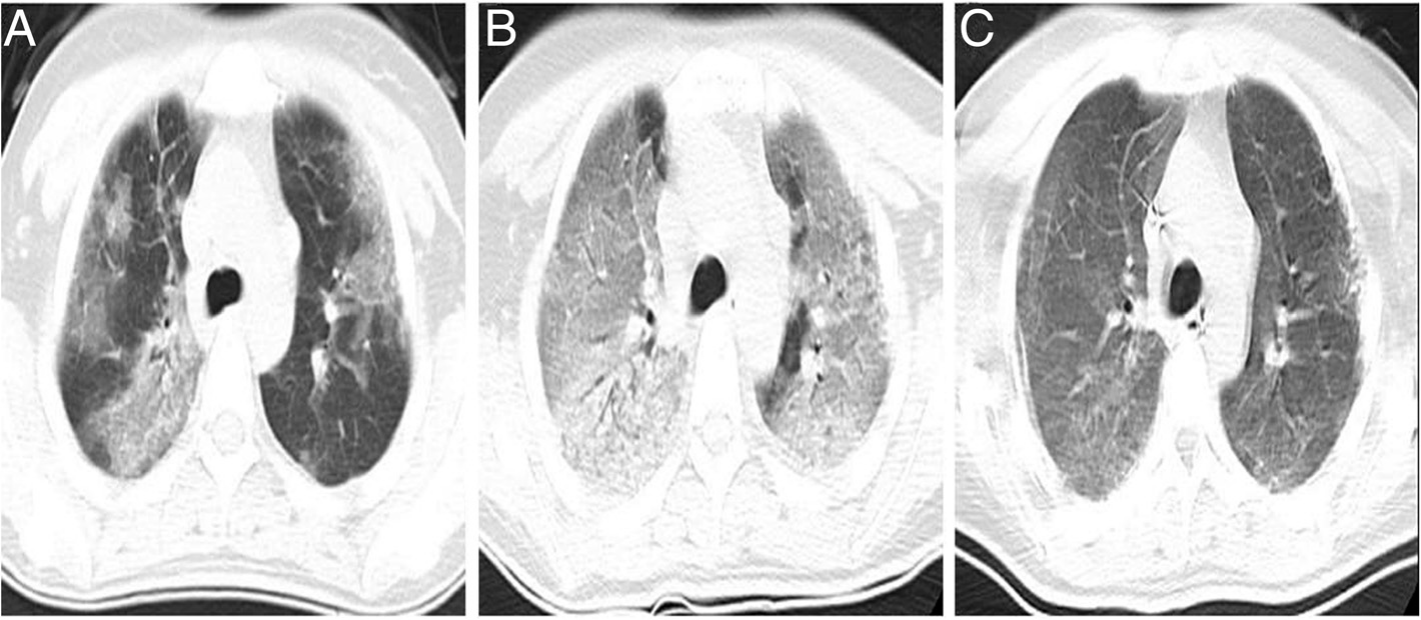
Radiological findings of the patient. A, Chest High-resolution computed tomography (HRCT) showed bilateral diffuse airspace opacification when the patient was hospitalized; B, Chest HRCT, taken on the fourth hospital day, demonstrated the worsening of diffused ground-glass opacification. C, the HRCT findings of the patient, who was treated with high dose of methylprednisolone for 5 d, showed diffuse ground-glass attenuation.
